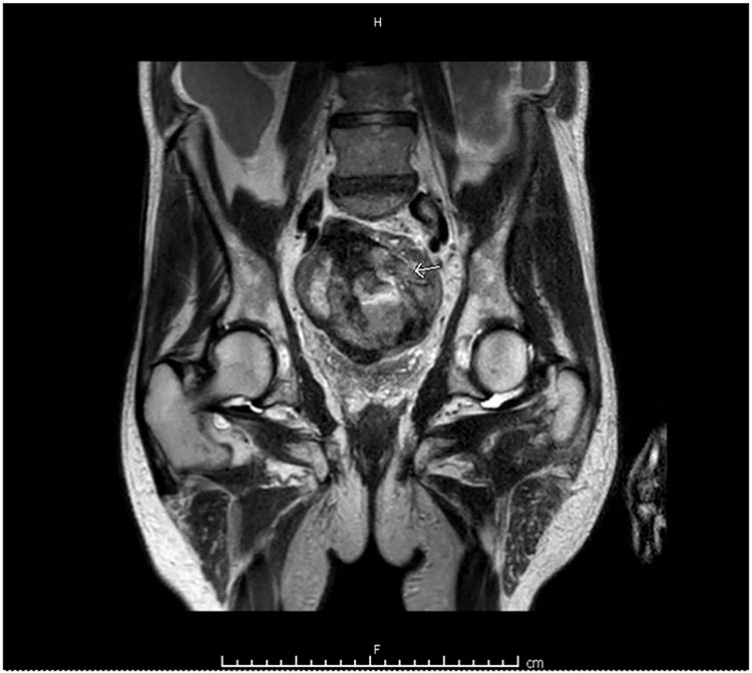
MRI abdomen and pelvis with contrast identified small bowel involvement in the lateral side wall of the hematoma within the pelvis.
